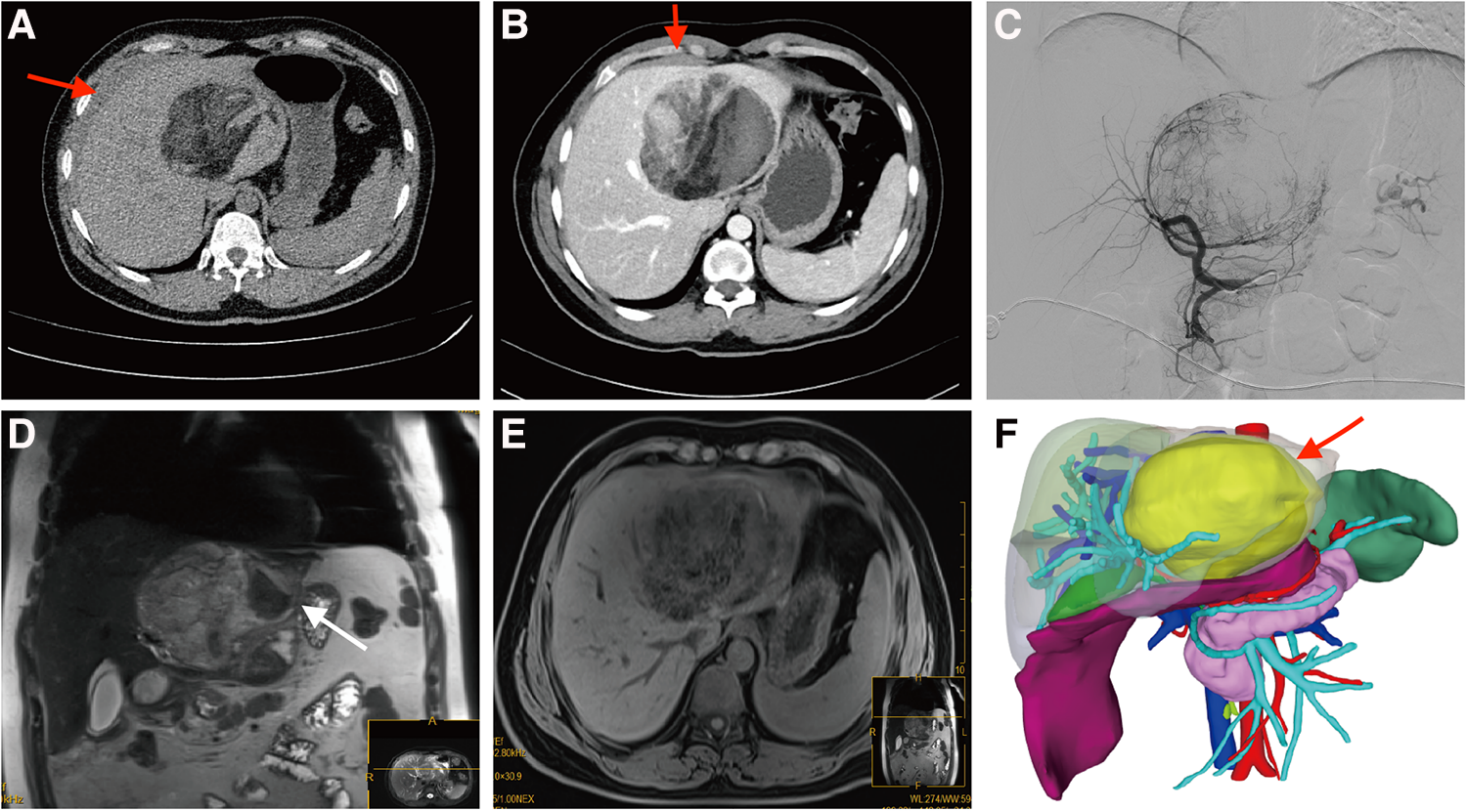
Preoperative imaging findings. (A,B) Abdominal computed tomography (CT) scans revealed a prominently sized mass with heterogeneous density located within the left hepatic lobe. In addition, intraabdominal bleeding (red arrow) was observed near the liver. The contrast-enhanced CT scan revealed heterogeneous enhancement within the lesion, which showed close adherence to the middle hepatic vein and the curvature of the stomach. (C) Angiography findings showed significant thickening and increased branching of the left hepatic artery, suggesting a robust blood supply to the tumor. (D,E) The hypointense signal observed on the MRI confirmed that the lesion was not consistent with a hepatocellular tumor. The white arrow indicated the rupture of the tumor. (F) 3D reconstruction model based on CT results. The purple area below the liver indicated blood accumulation. The yellow arrow indicated the tumor.
